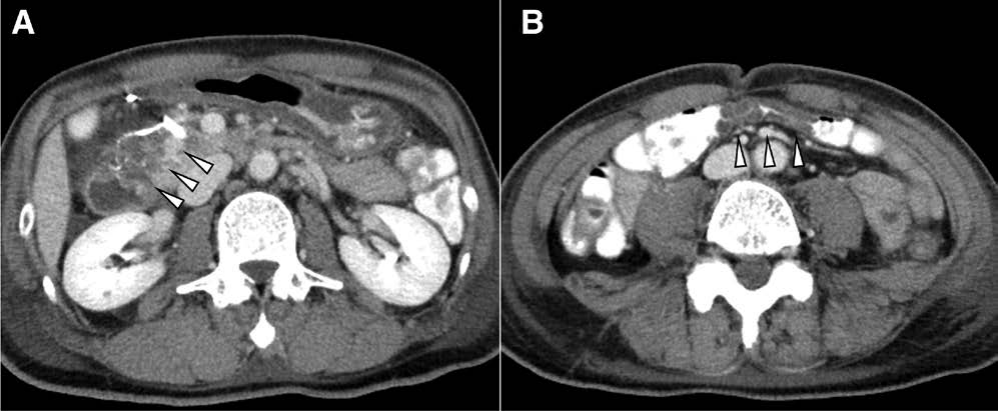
Contrast-enhanced computed tomography conducted 5 days after interventional radiology showed (A) diminished contrast effect in the pancreaticoduodenal region (arrowheads), and (B) partial edema and stenosis of the transverse colon (arrowheads).
